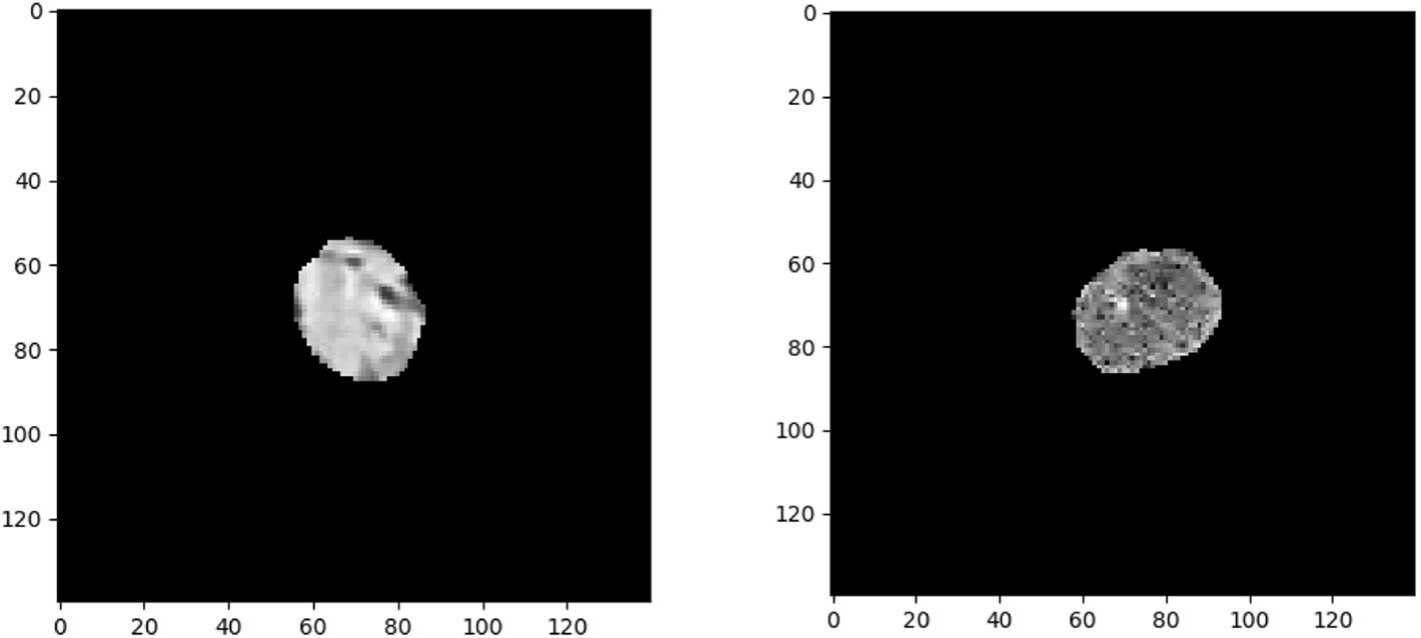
Example of the input CT images. Left: NSCLC tumor from Cohort 1. Right: PDAC tumor from Cohort 2
